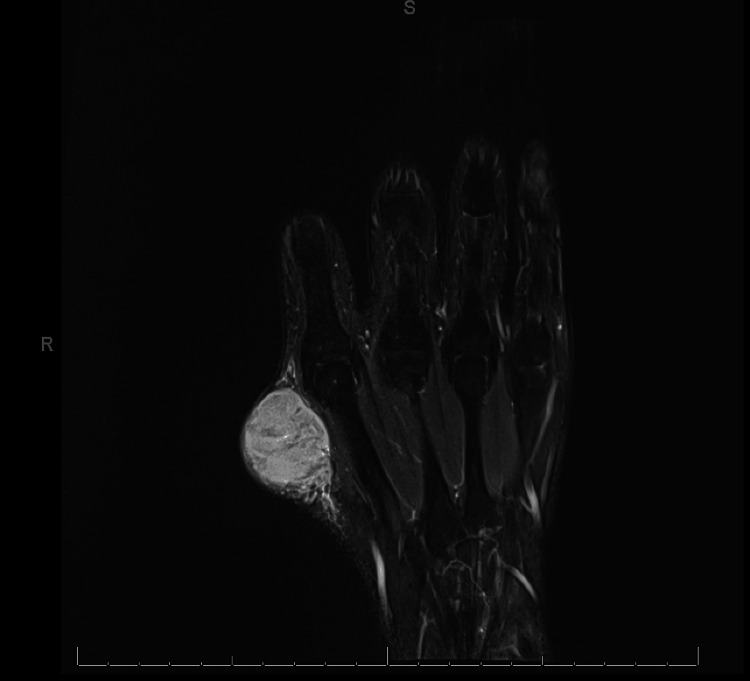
T2-weighted MRI of the right hand without contrast demonstrating heterogeneously increased T2 signal without high-flow vasculature
